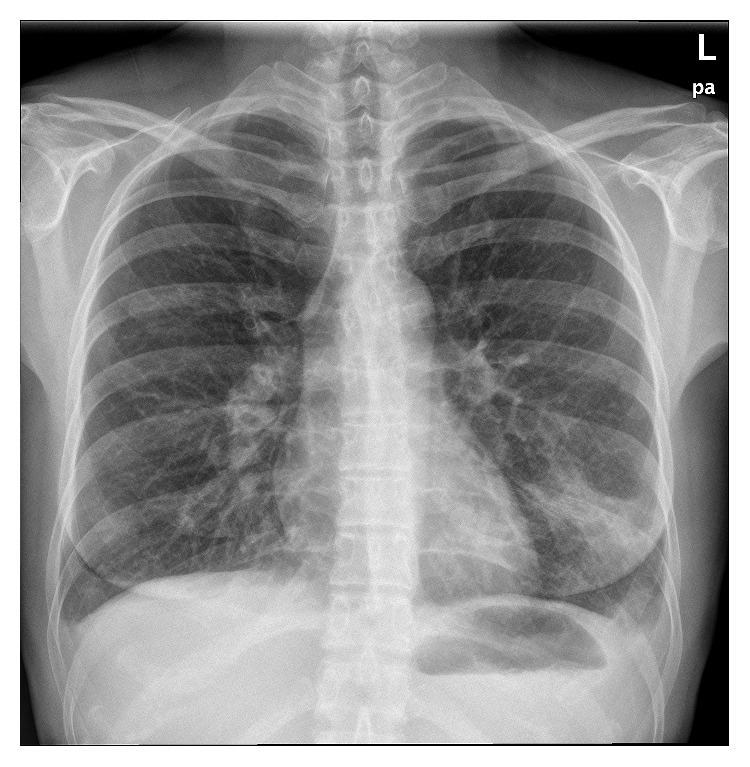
Chest X-ray at the patient's exit.
